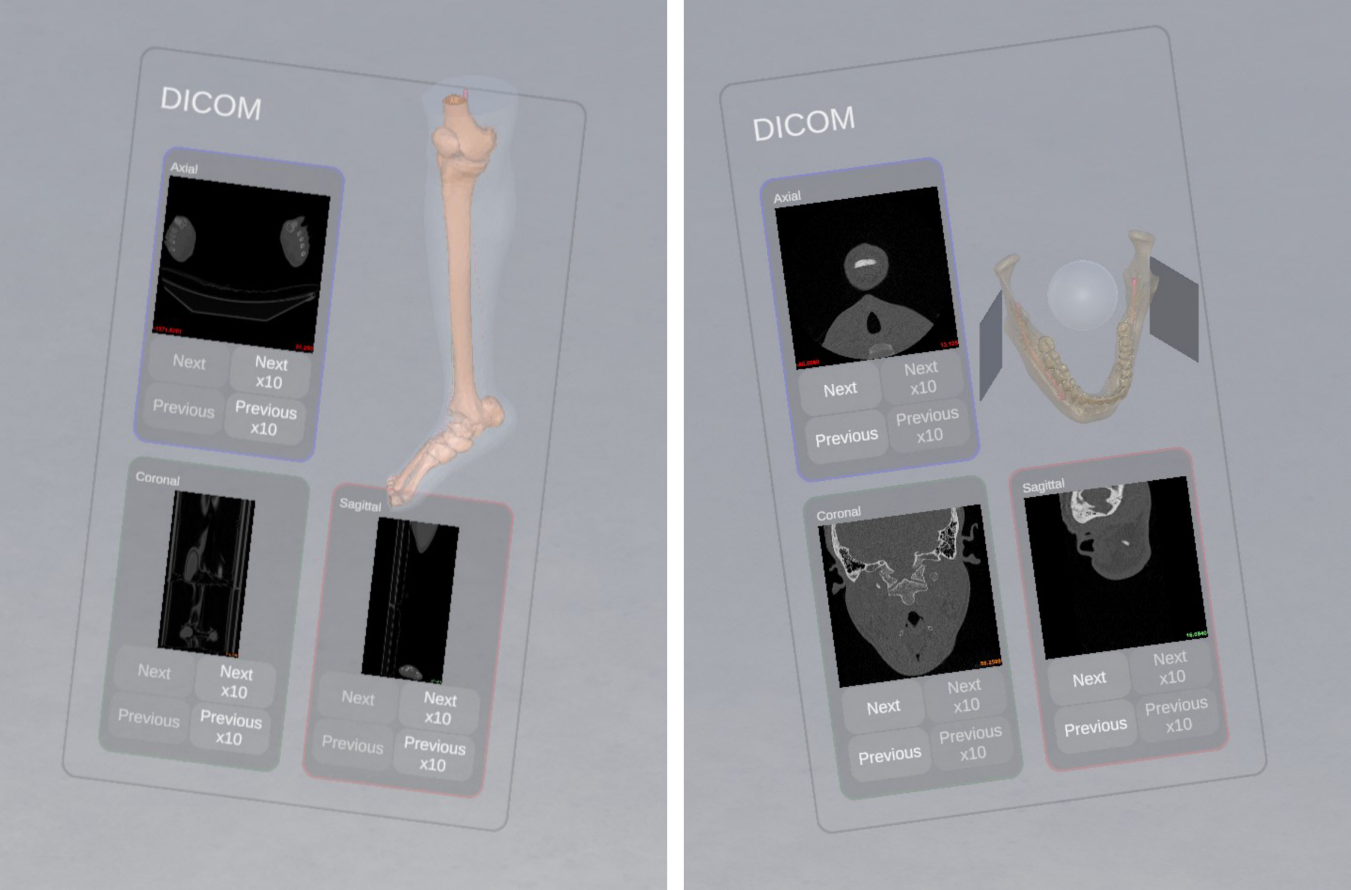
CT scans in axial, coronal, and sagittal plans and 3D model of the mandible (left) and the fibula (right).
